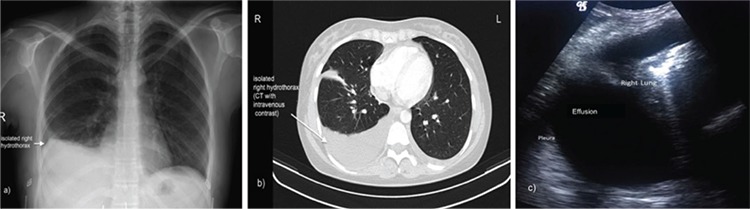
The imaging of isolated pleural effusion under X-ray (a), computed tomography (b), and (c) ultrasonography
